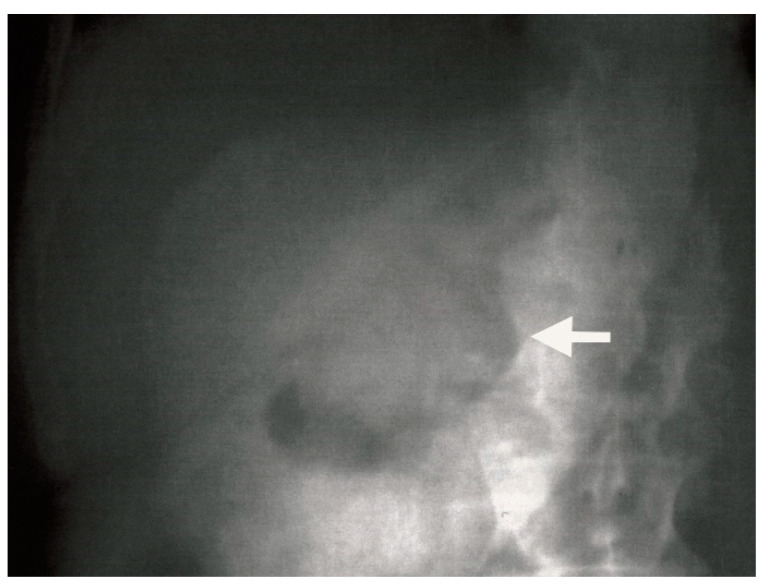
Plain abdominal radiograph showing pneumobilia (white arrow) in the gallbladder.
